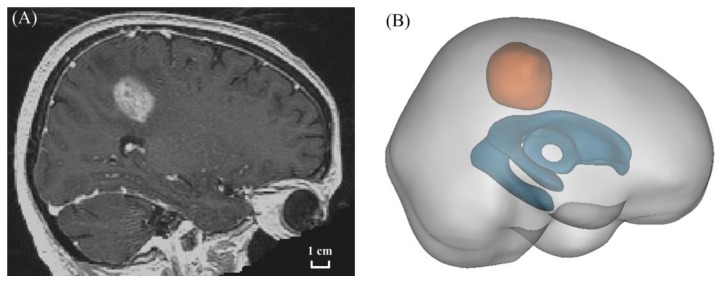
Model geometry. (A) MR image, and (B) reconstructed 3D geometry of brain tumour (orange) and its surrounding normal tissue (grey). The brain ventricle is coloured in cyan.
